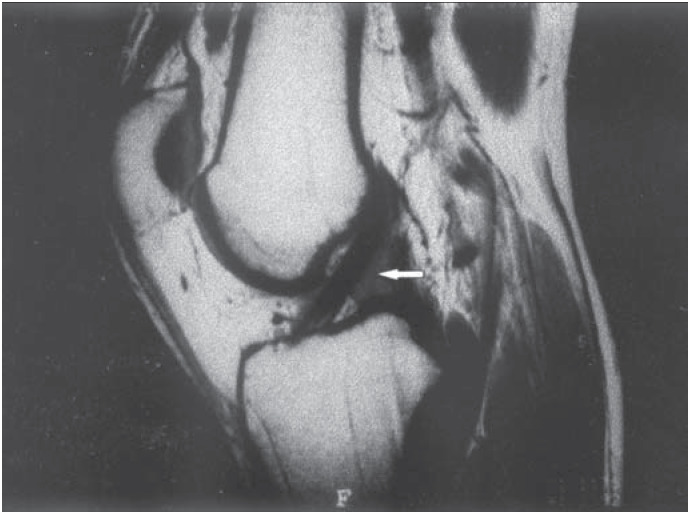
Magnetic resonance imaging of the patient's left knee. White arrow shows the anterior cruciate ligament ganglion.
